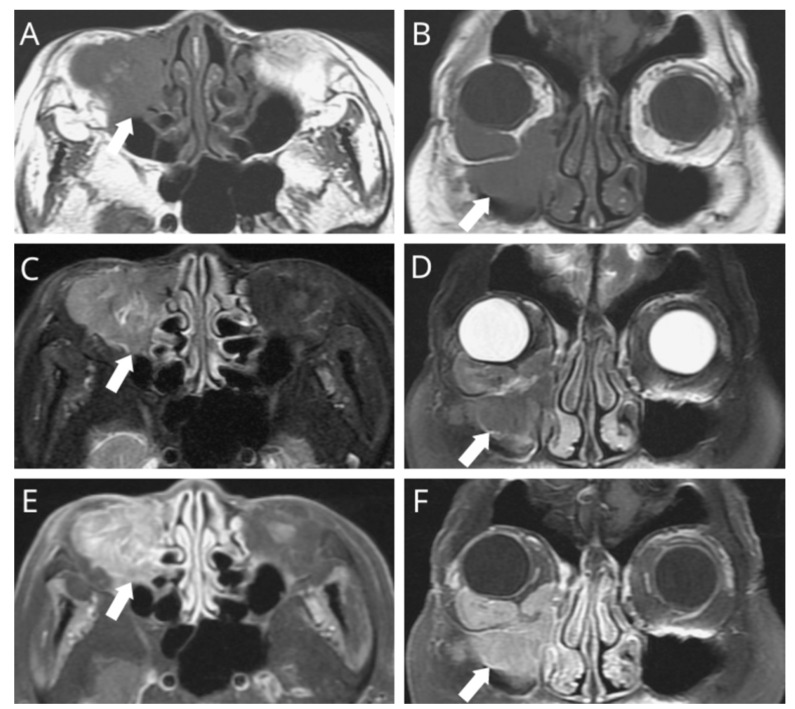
A 71-year-old woman visited our clinic due to right medial orbital swellings associated with limited ocular mobility, drooping eyelids, blurred vision, and persistent tearing in the previous six months. On further ophthalmic testing, there was a grade-3 infraversion oculus dexter, but a naso-endoscopy demonstrated negative findings. ((A,B) arrow denotes the primary tumor) A subsequent MRI revealed a lesion of irregular contour presenting with homogenous hypointense signals on the T1-weighted sequence, with an associated tumor growth measuring 4.2 cm. ((C,D) arrow denotes the primary tumor) The sagittal and coronal fat-saturated T2-weighted sequence revealed the lesion location within the lacrimal sac, extending into the right maxillary sinus and orbit, causing prominent bony destruction. ((E,F) arrow denotes the primary tumor) The fat-saturated T1-weighted MRI found a vivid heterogeneous enhancement upon contrast administration.
